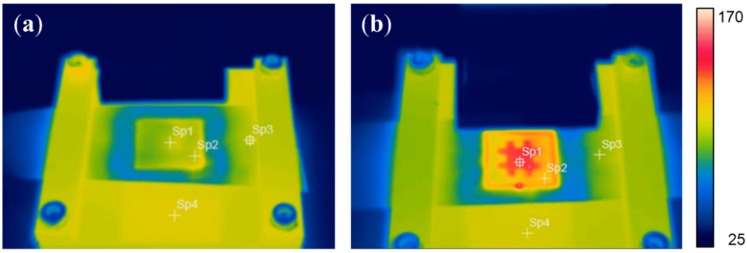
Temperature distributions after ultrasonic imprinting: (a) normal imprinting; and (b) selective imprinting.
